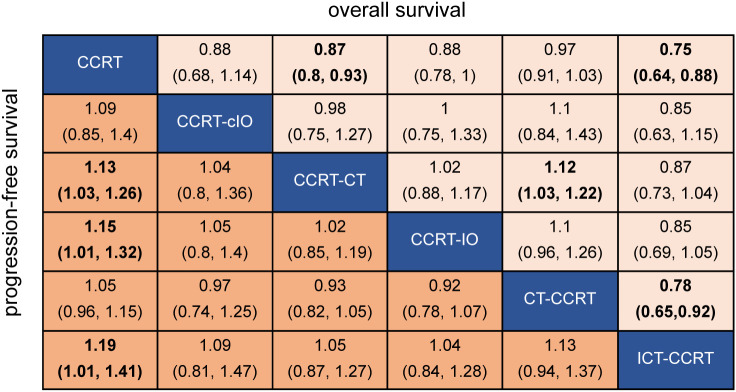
Efficacy of the 6 treatment regimens in network meta-analysis. Data are pooled HR (95% CI). Bold data indicate a significant difference. HR, hazard ratio; CI, confidence interval; CCRT, concurrent chemoradiotherapy; ICT-CCRT, induction immunochemotherapy plus CCRT; CCRT-cIO, CCRT plus concurrent immunotherapy; CCRT-IO, CCRT plus consolidation immunotherapy; CT-CCRT, induction chemotherapy plus CCRT; CCRT-CT, CCRT plus consolidation chemotherapy.
